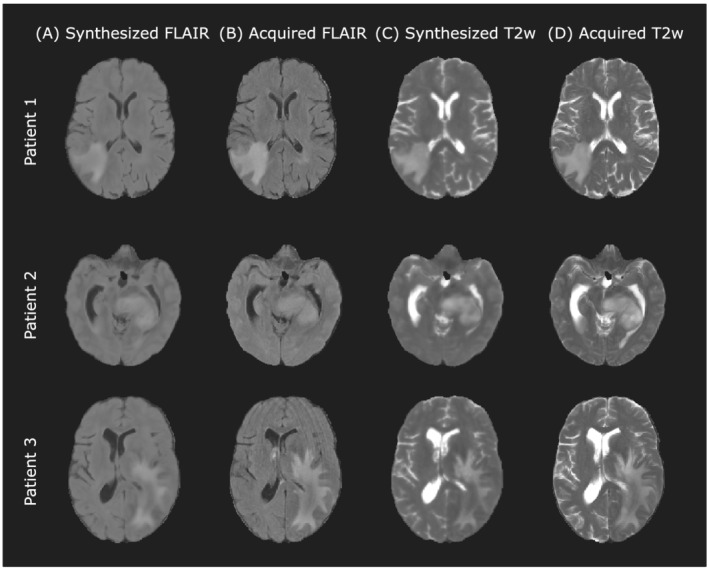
A representative axial slice of the images synthesized by the self‐supervised CNN for different test patients of Dataset24. A, Synthesized FLAIR images. B, Corresponding actually acquired FLAIR images. C, Synthesized T2w images. D, Corresponding actually acquired T2w images
